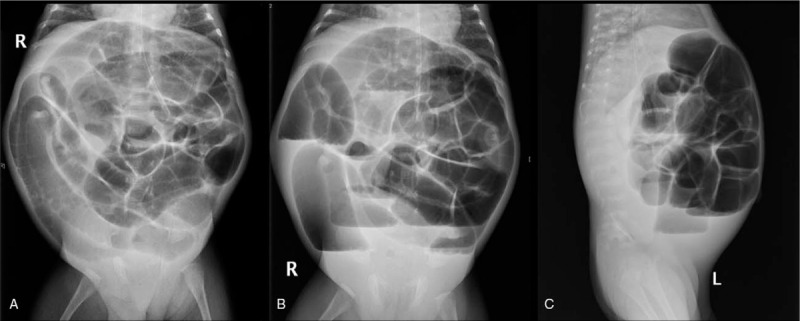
Abdominal X-ray appearance of a post-NEC stricture. A: Intestinal expansion in a decubitus film. B: Intestinal expansion and liquid level in a stand-up film. C: Fluid and dilatation of the lower ileus in a lateral film.
